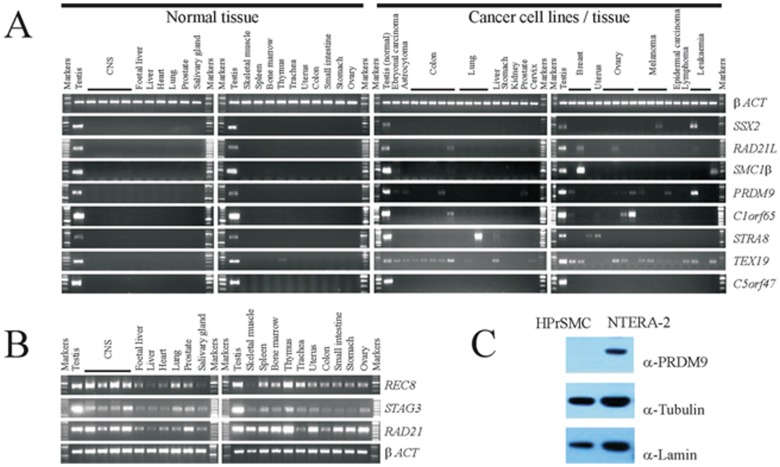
Examples of gene expression and protein production profiles for predicted meiCT genes A. Agarose gels showing RT-PCR profiles generated from a range of normal human tissues obtained *post mortem* (left two panels) and cDNA generated from a range of cancer cell lines or solid tumours (right hand two panels). The expression profile for *betaACT* is a positive control (top row). The profile for *SSX2* provides an example of a previously characterised X-CT gene. Five examples of testis-restricted meiCT genes are shown (*RAD21L/SMC1beta/PRDM9/C1orf65/STRA8*) along with the expression profile of one testis selective meiCT gene, *TEX19*. The *C5orf47* profile provides an example of genes which were testis-restricted with no evidence of expression in any of the cancer cells tested. B. Agarose gels showing RT-PCR profiles for normal human tissues for the mitotic cohesion gene *RAD21* and the two cohesin genes *REC8* and *STAG3*. C. Western blots showing the presence of the PRDM9 protein in the cancer cell line NTERA-2, but not in primary cultures of human prostate smooth muscle cells (HPrSMC).
